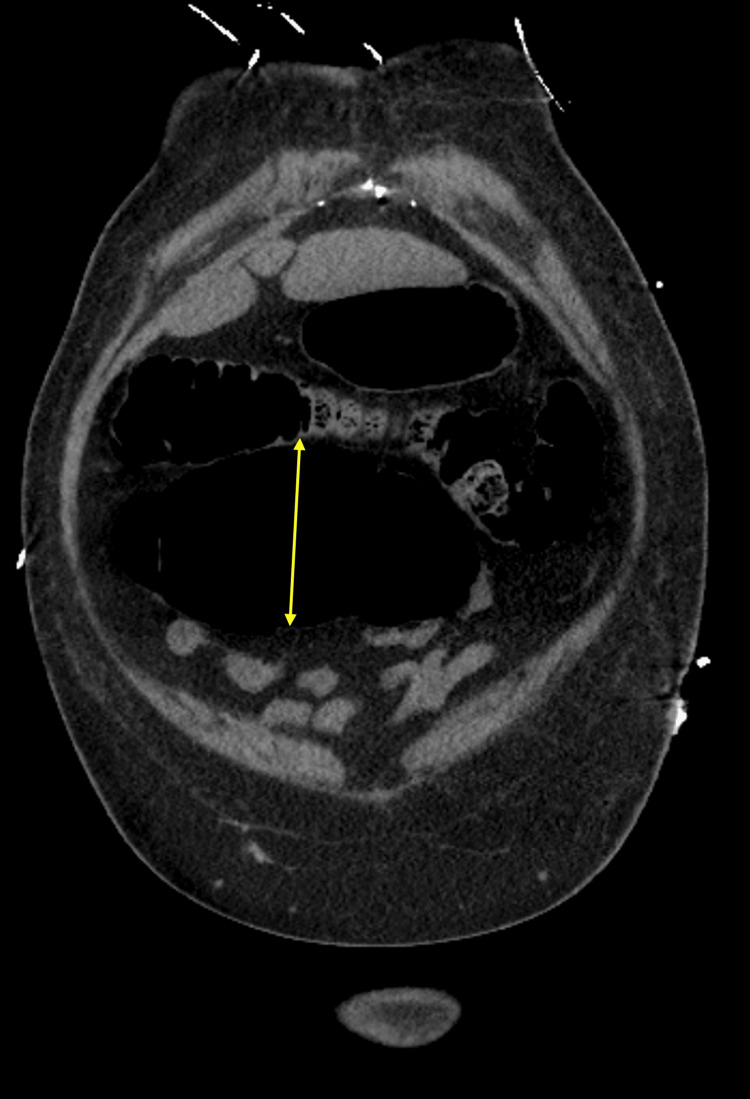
Computed tomography image Coronal image from a computed tomography scan of the abdomen showing a dilated cecum (9.3 cm, arrow) with an anteromedial orientation, concerning for cecal bascule.
